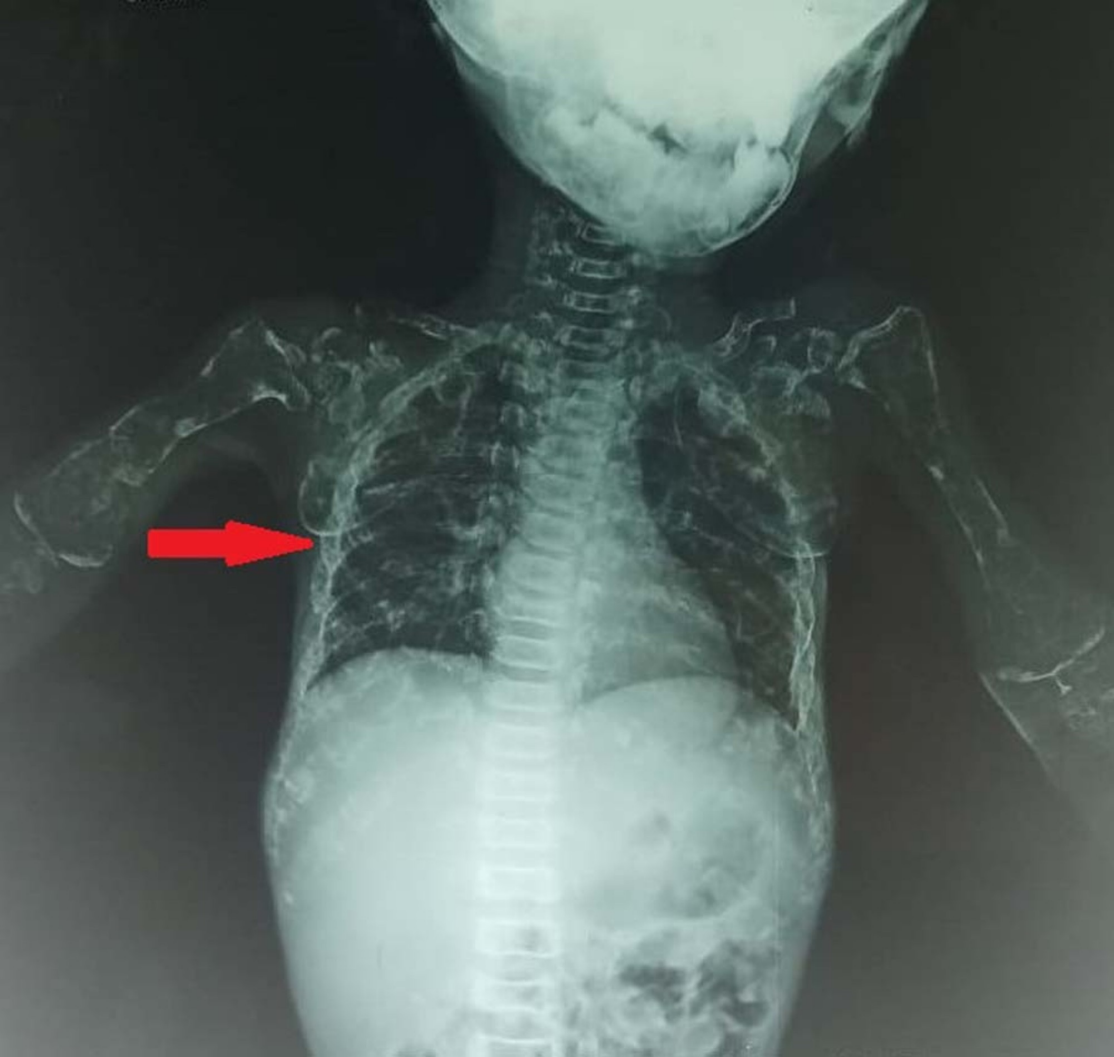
Chest X-ray showing excessive amount of multiple rib fractures.
